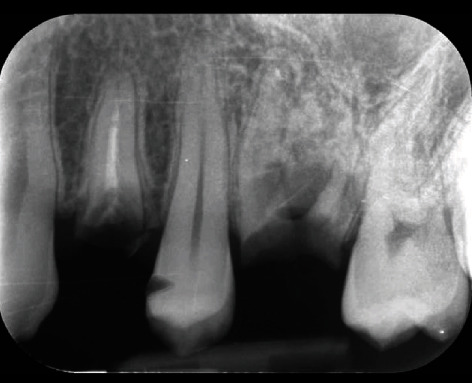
X-ray of the premolar with the presence of residual roots and the cavity on the crown.
